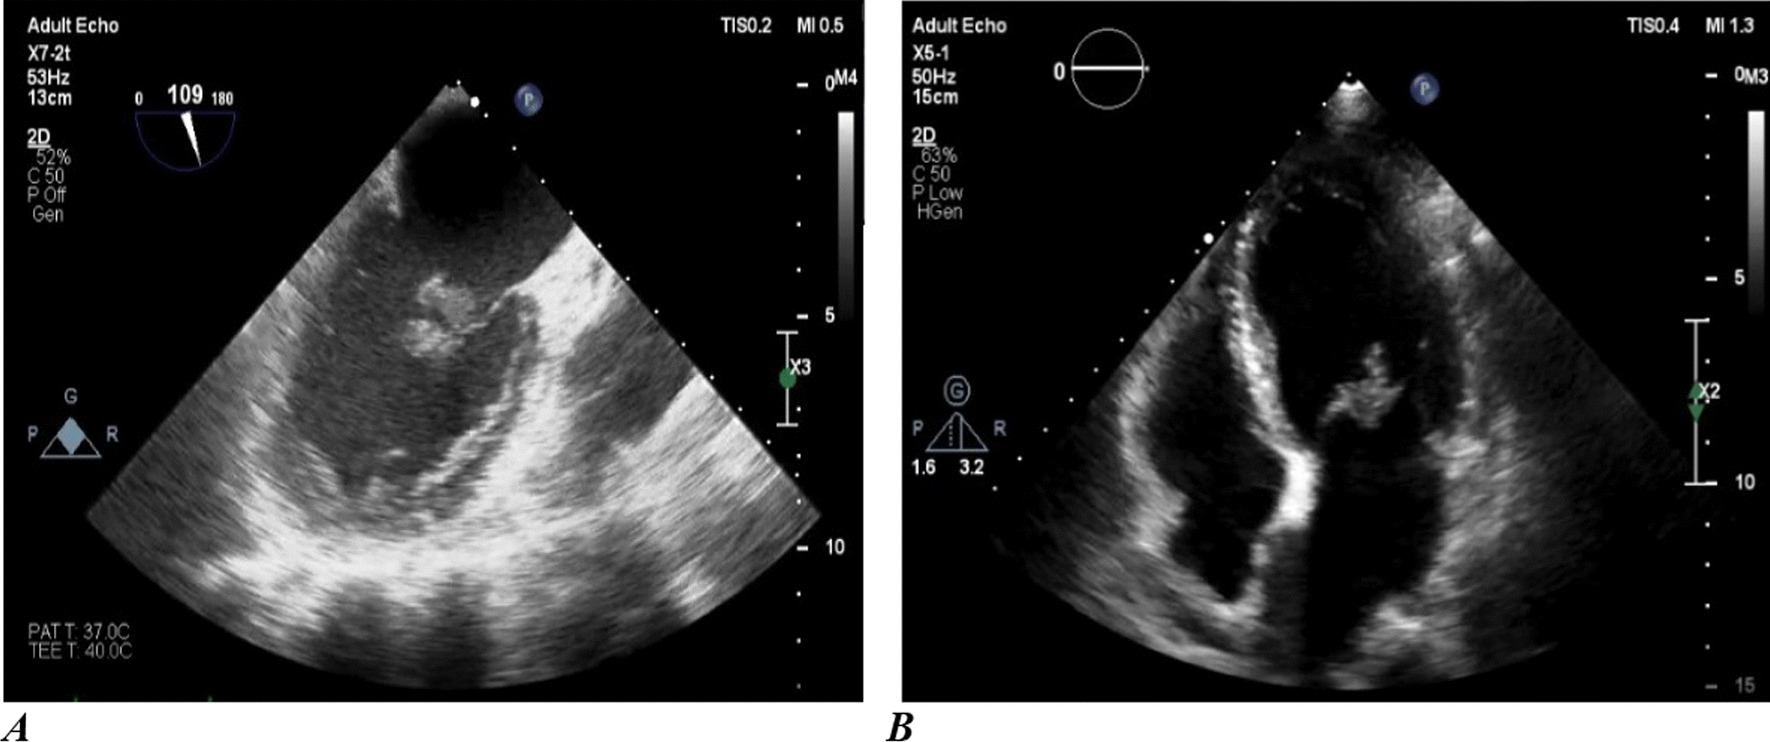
Echocardiogram of the patient showing a large mobile mass on tip of A2 scallop (with a length of roughly 16 mm) and another mass (6 mm × 7 mm) attached to mitral valve chorda in the left ventricle. Left picture a is a transesophageal echo in a 109-degree view, and the right picture b is a transthoracic echo in a four-chamber view
